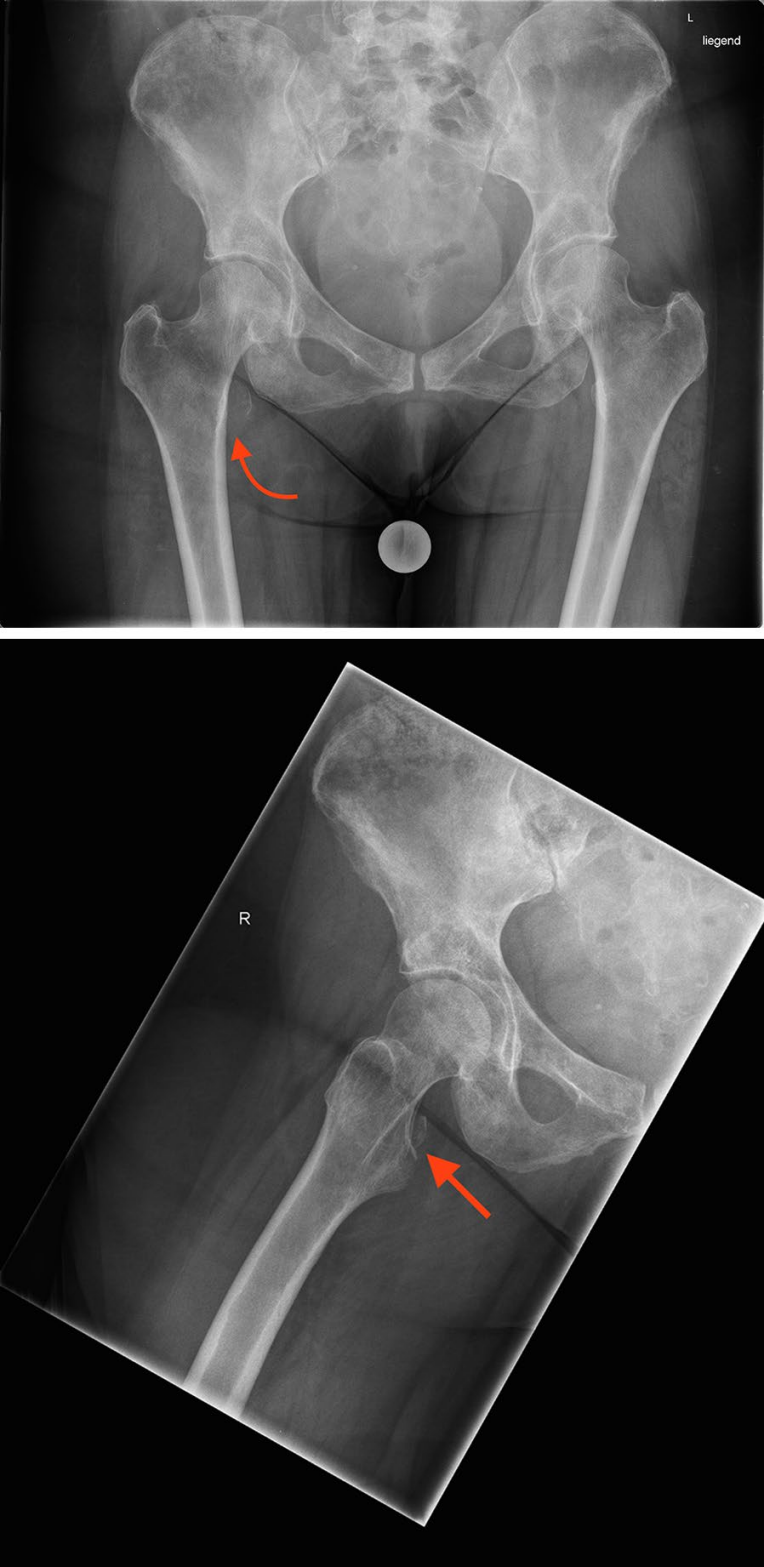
X-ray of the pelvis in AP view shows an isolated lesser trochanteric fracture (see *red arrows*) beside multiple osteolytic processes of the pelvis
